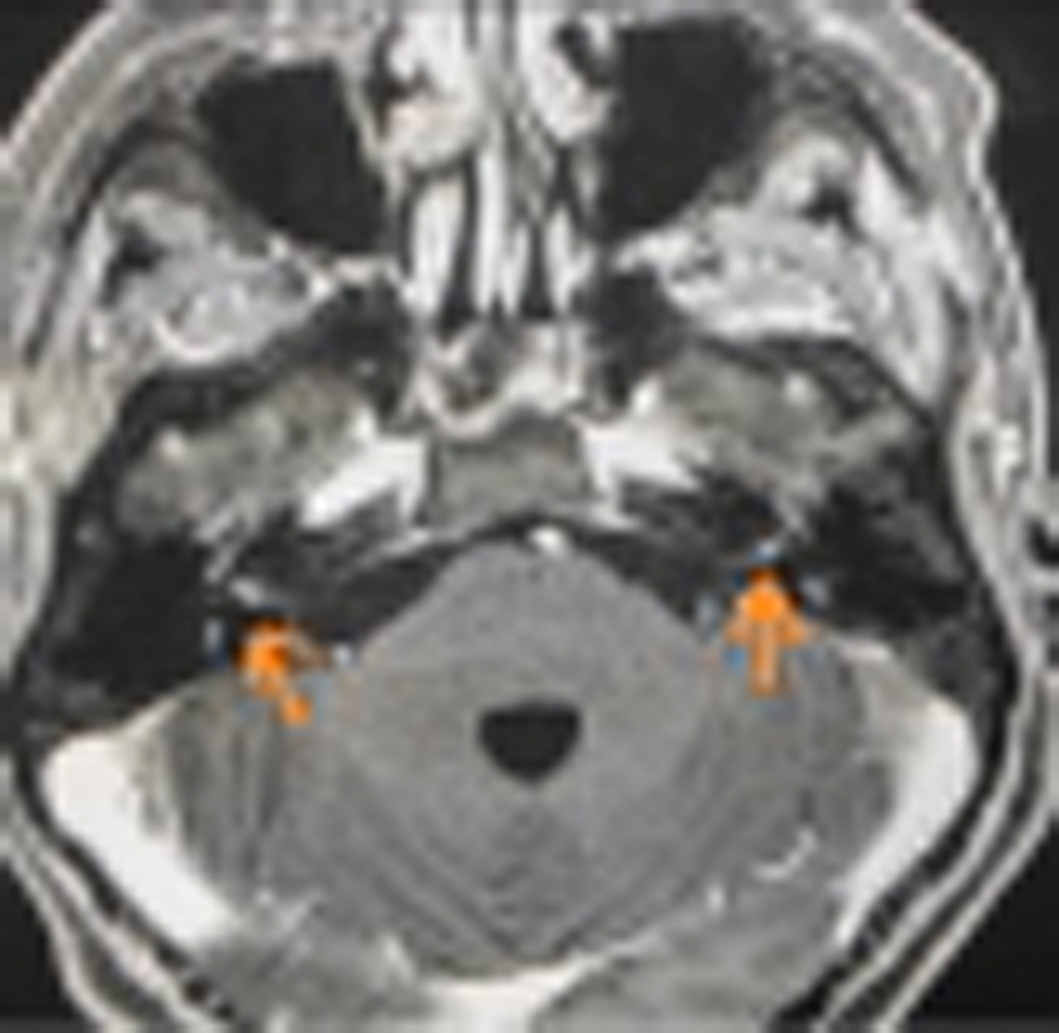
MRI with gadolinium for the study of internal acoustic canal (IAC) and cerebellopontine‐angle cistern. Arrows points the symmetrical and pathological contrast enhancement of the distal portions of the intracanalicular tract of the facial nerves.
